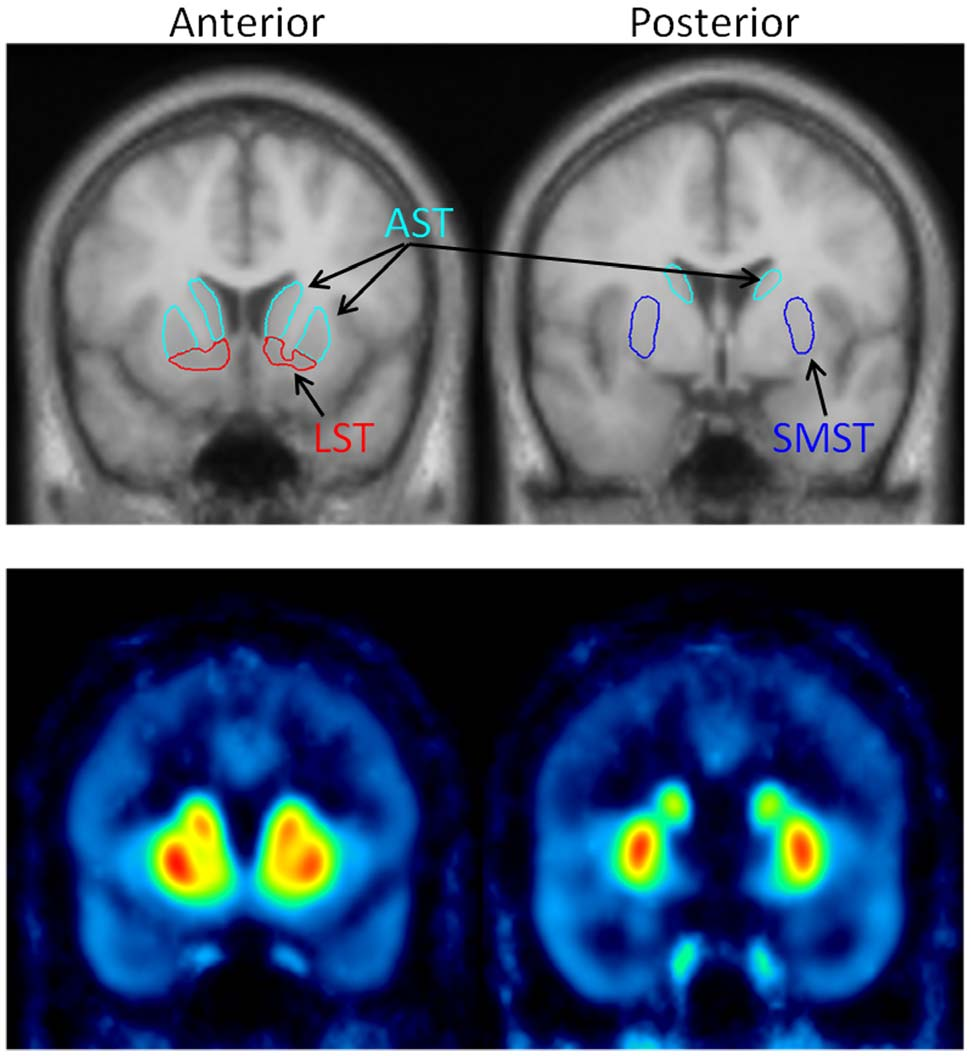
Definition of striatal functional subdivisions. Upper panel: MR images and regions of interest. Lower panel: parametric images corresponding to MR images in the upper panel.
